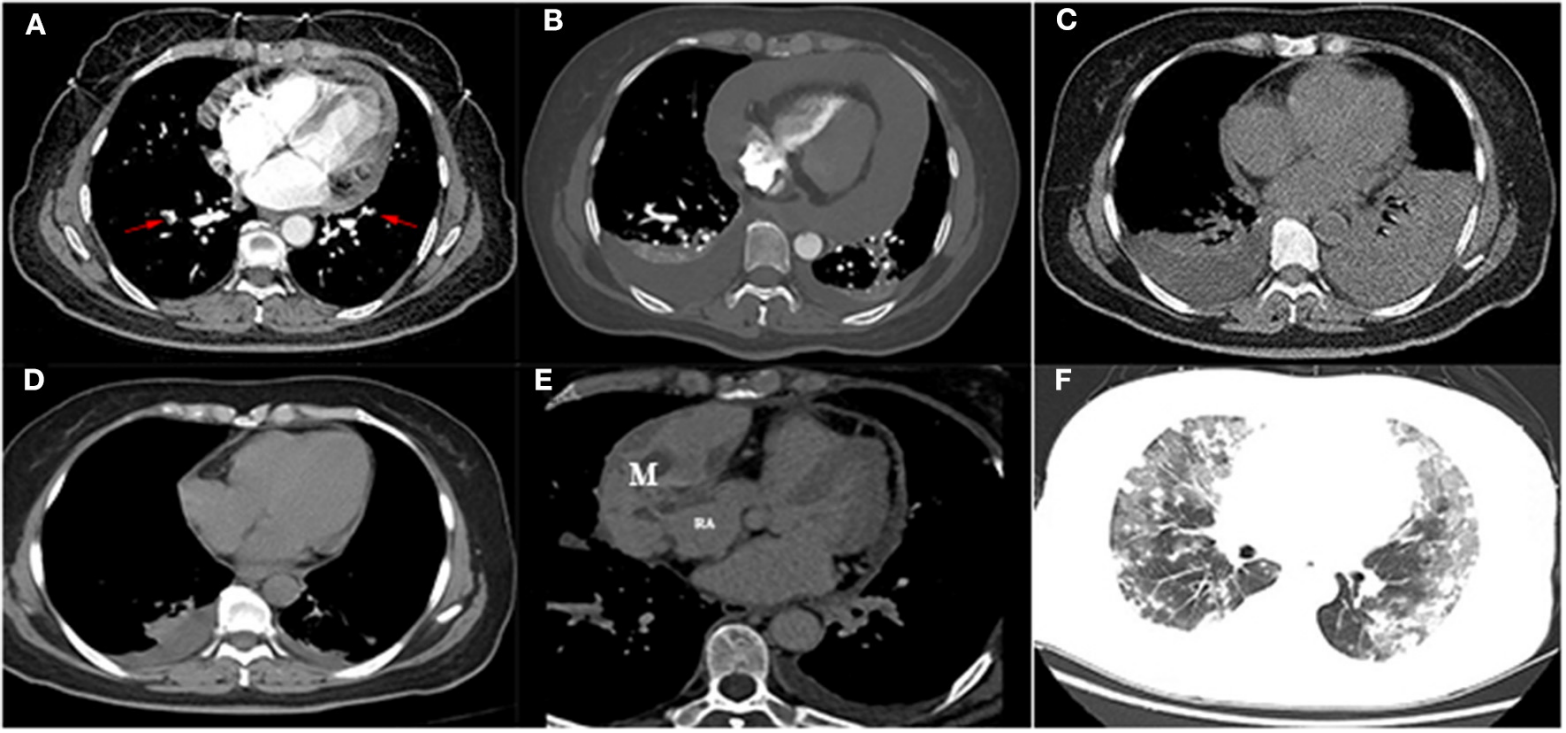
(A) Computed tomography pulmonary angiography showed multiple embolisms of bilateral pulmonary arteries (arrow) with a mild-sized pericardial effusion. (B) Computed tomography showed a severe-sized pericardial effusion and bilateral pleural effusion after heparin anticoagulation. (C) The left pleural effusion was increased after pericardial drainage. (D) Computed tomography revealed mild bilateral pleural effusion and pericardial effusion after drainage. (E) Contrast-enhanced computer tomography showed a mixed mass located in the right pericardium (4.5 × 8.5 cm), and the boundary was unclear between the mass and the right atrial wall, heterogeneous thickened pericardium, and moderate pericardial effusion locally. (F) Multiple metastatic nodules surrounded by a wide range of ground-glass-like effusion (diffuse alveolar hemorrhage) were identified in the bilateral lungs. M, mass; RA, right atrium.
